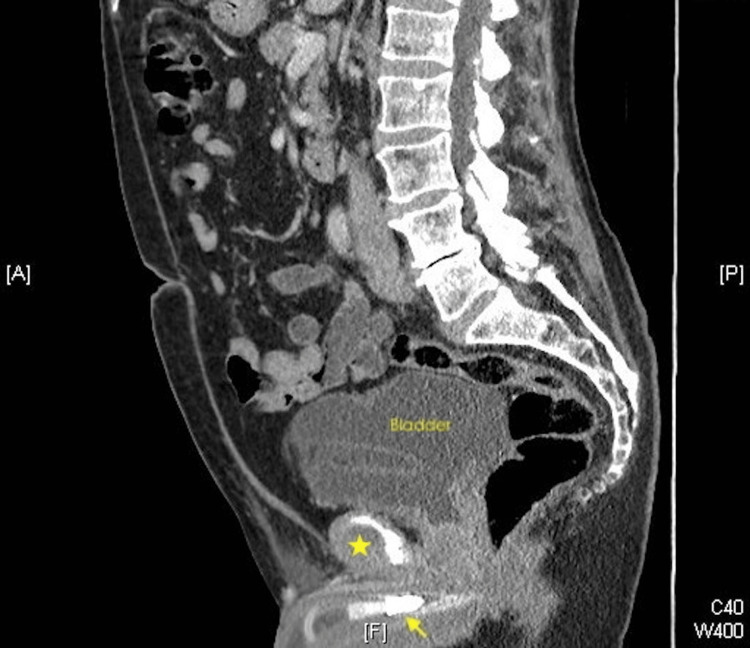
Computed tomography (sagittal view) scan demonstrating penile prosthesis (yellow arrow), and a loculated fluid collection (star).
